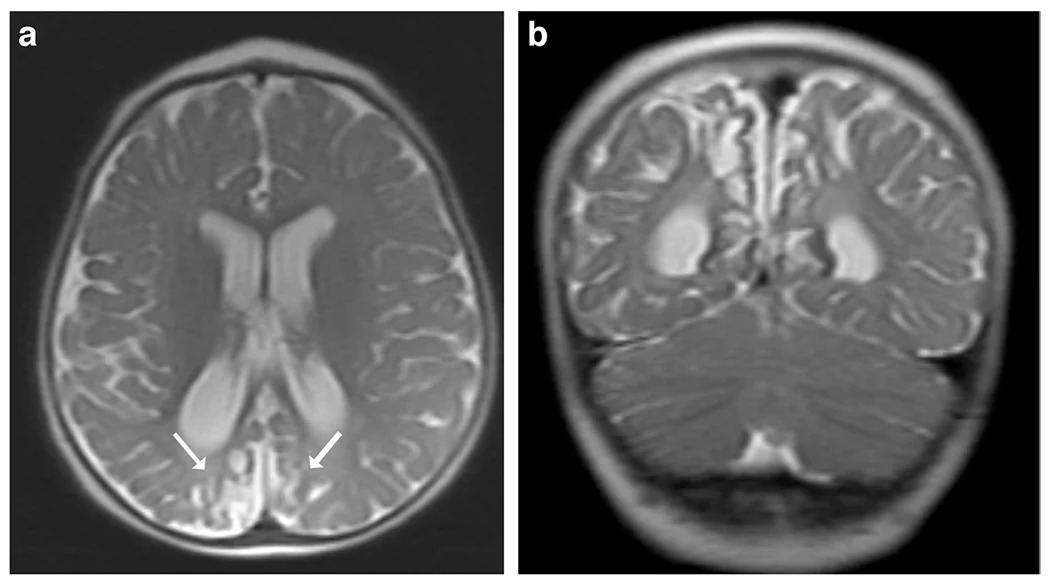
Eleven-month-old male infant with a history of neonatal hypoglycemia. Single-shot fast-spin echo T2-weighted (a) axial and (b) coronal limited brain magnetic resonance imaging have evidence of occipital cortical or subcortical volume loss, characterized by volume loss and gliosis (*arrows* in a)
